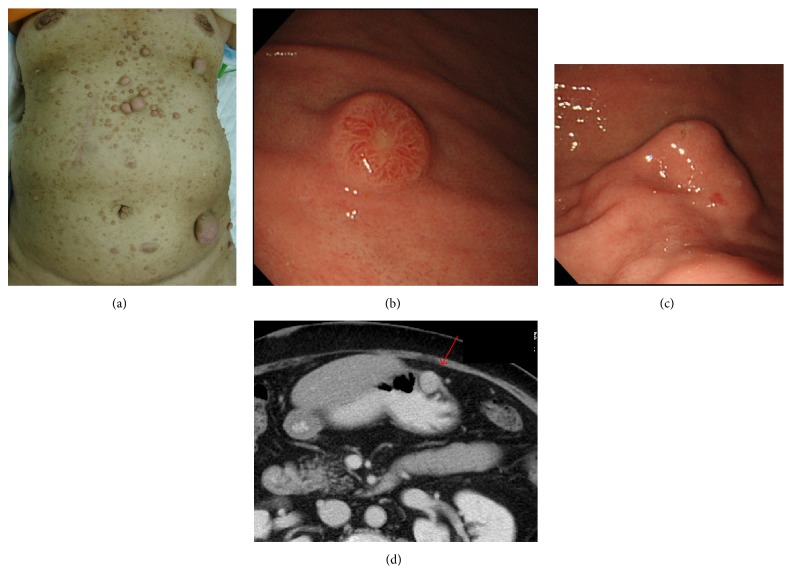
(a) There are multiple neurofibromas and some cafe-au-lait spots all over the body skin. (b) Upper gastrointestinal endoscopy shows neuroendocrine tumor located on posterior side of upper gastric wall. The size was about 10 mm. (c) And submucosal tumor located on greater curvature side of middle gastric wall. The size was about 30 mm. (d) Computed tomography showed only a SMT (arrow); the size was about 30 mm and the surface was smooth. Other lesions could not be pointed out.
